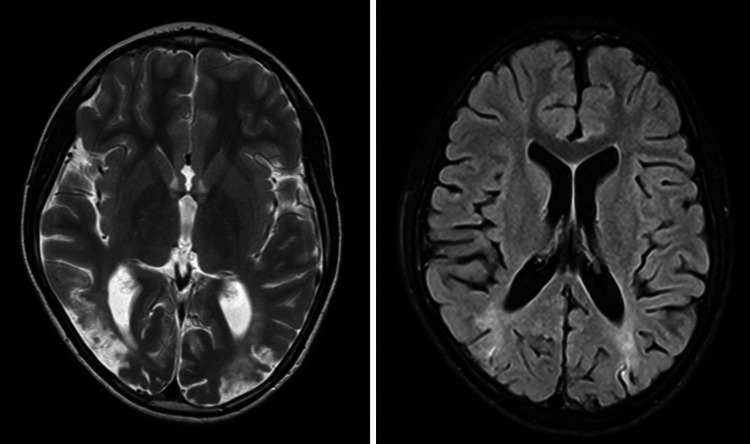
Brain magnetic resonance imaging, one year after PRES diagnosis. The remaining focal areas of hyperintense signal in the bilateral parieto-occipital white matter in T2 and FLAIR sequences were reduced when compared to admission imaging. PRES, posterior reversible encephalopathy syndrome; FLAIR, fluid-attenuated inversion recovery.
